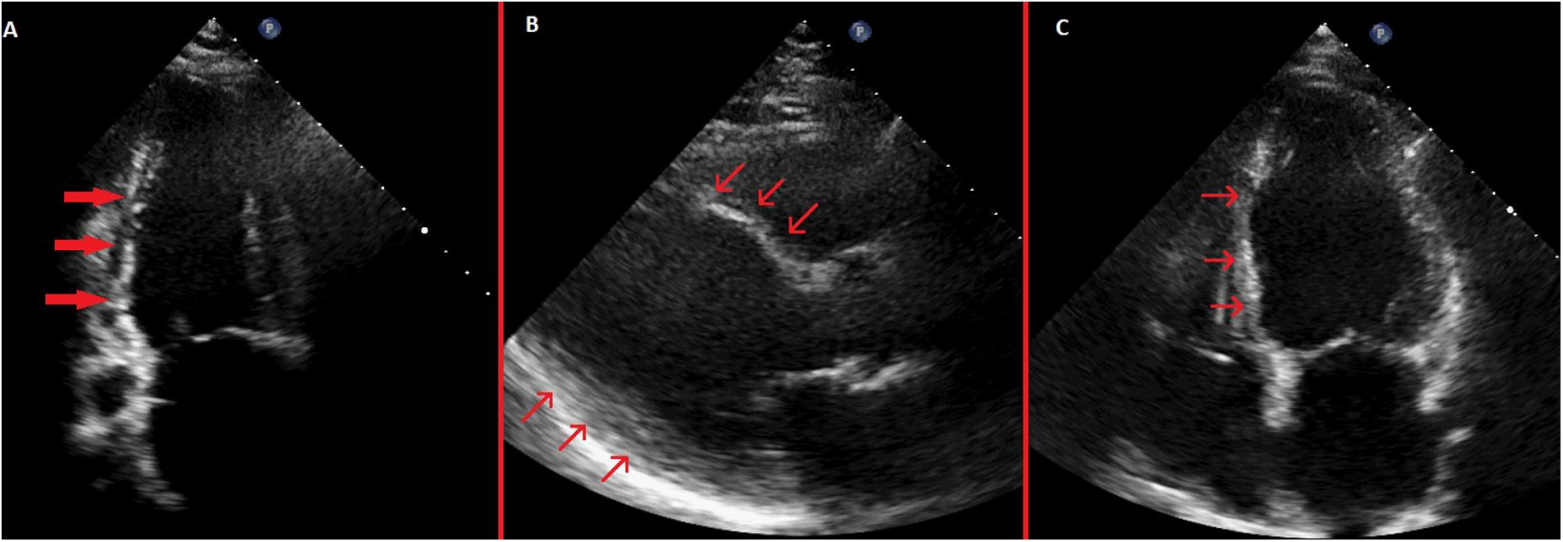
Pre-discharge transthoracic echocardiography images. (A) Systolic apical 2-chamber view showing severe hypokinesis of the inferior wall with thinning of the inferior wall myocardium. (B) Systolic parasternal long-axis view showing akinetic and thinned anterior septum and inferolateral wall hypokinesis. (C) Systolic apical 4-chamber view showing akinetic and thinned basal and mid septum.
